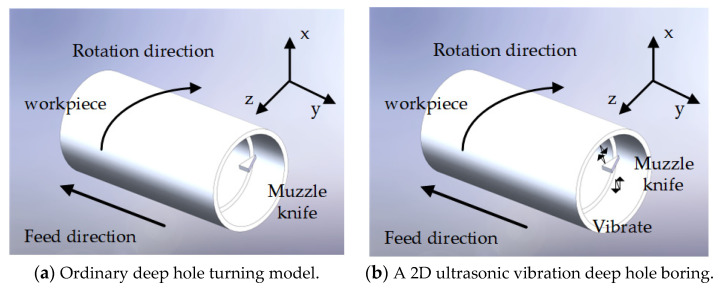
Model of Ultrasonic Vibration Turning of Inner Holes.
